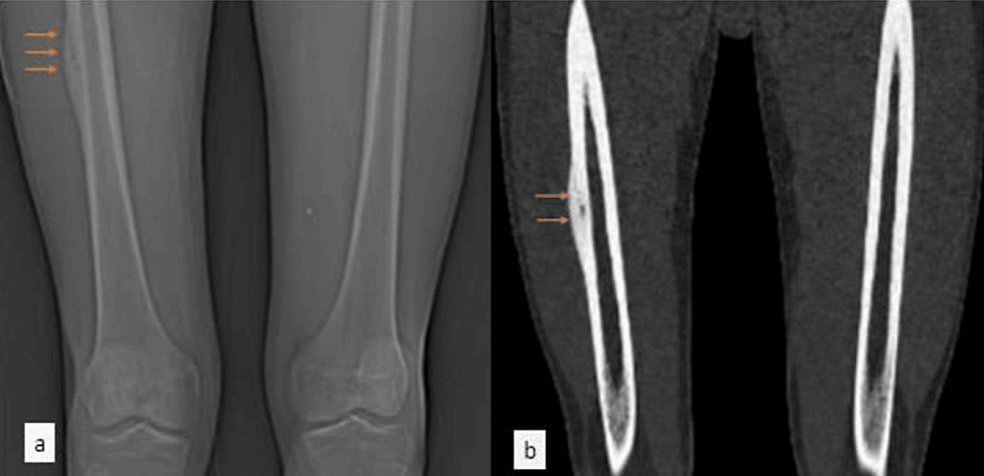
CT of the bilateral femur coronal sections of the topogram (a) and bone window (b) showing cortical-based lesion with a central osteolytic nidus suggesting OO CT, computed tomography; OO, osteoid osteoma
